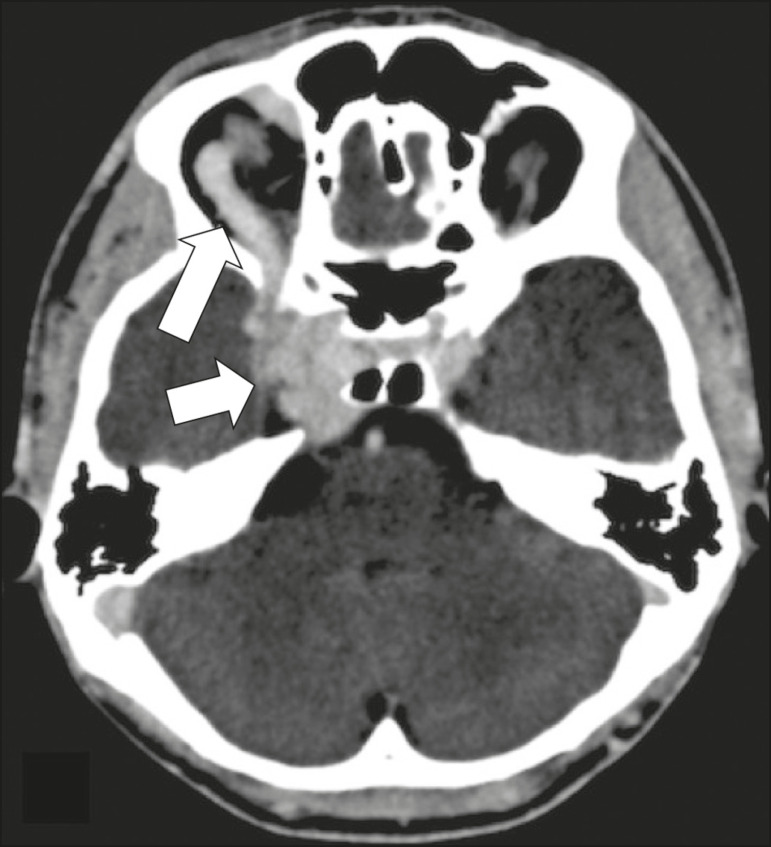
Carotid-cavernous fistula. Axial CT angiography showing dilation and early enhancement of the cavernous sinus and superior ophthalmic vein, resulting in right exophthalmia and convergent strabismus due to abducens nerve compression
